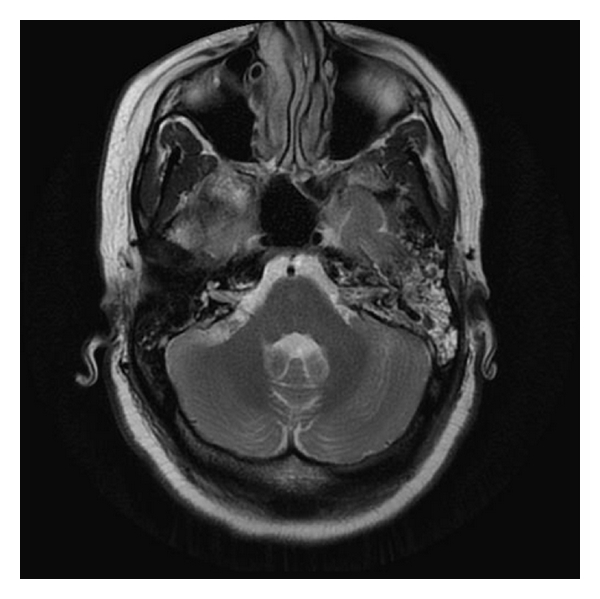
MRI of brain with contrast demonstrating fluid and enhancement within the mastoids, more extensive on the left, suggestive of mastoiditis.
